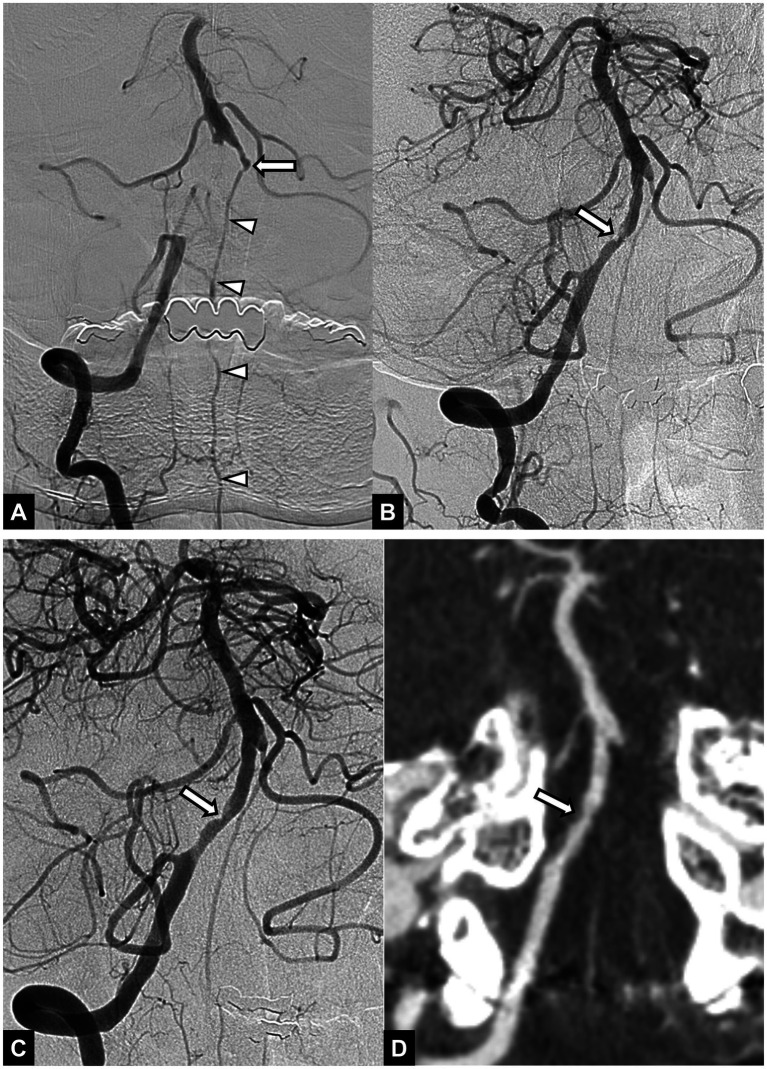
A 54-year-old male patient with acute stroke due to vertebral artery occlusion. (A) Initial angiogram shows an occlusion at the intracranial segment of the right vertebral artery. Basilar artery is opacified via collateral circulation from the anterior spinal artery (arrowheads). Note the chronic occlusion in the left vertebral artery (arrow). (B) Angiogram obtained after mechanical thrombectomy with a stent retriever shows successful recanalization with underlying focal irregular stenosis (arrow) in the right vertebral artery. (C) Final angiogram obtained after intracranial angioplasty shows successful reperfusion of the posterior circulation and remained stenosis (arrow) in the right vertebral artery. (D) Follow-up CT angiography obtained 48 h after endovascular treatment shows patent right vertebral artery with remained mild stenosis (arrow). The 3-month mRS score was 1.
